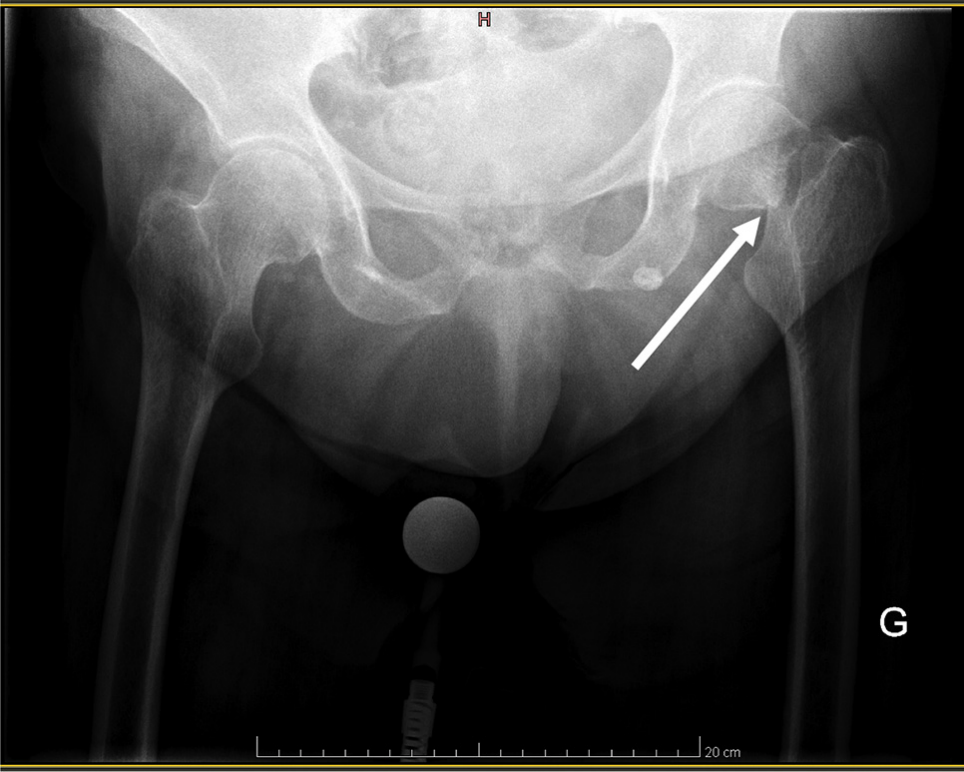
Plain radiography with anteroposterior pelvic projection showing a Garden IV left hip fracture with complete displacement (*white arrow*).
